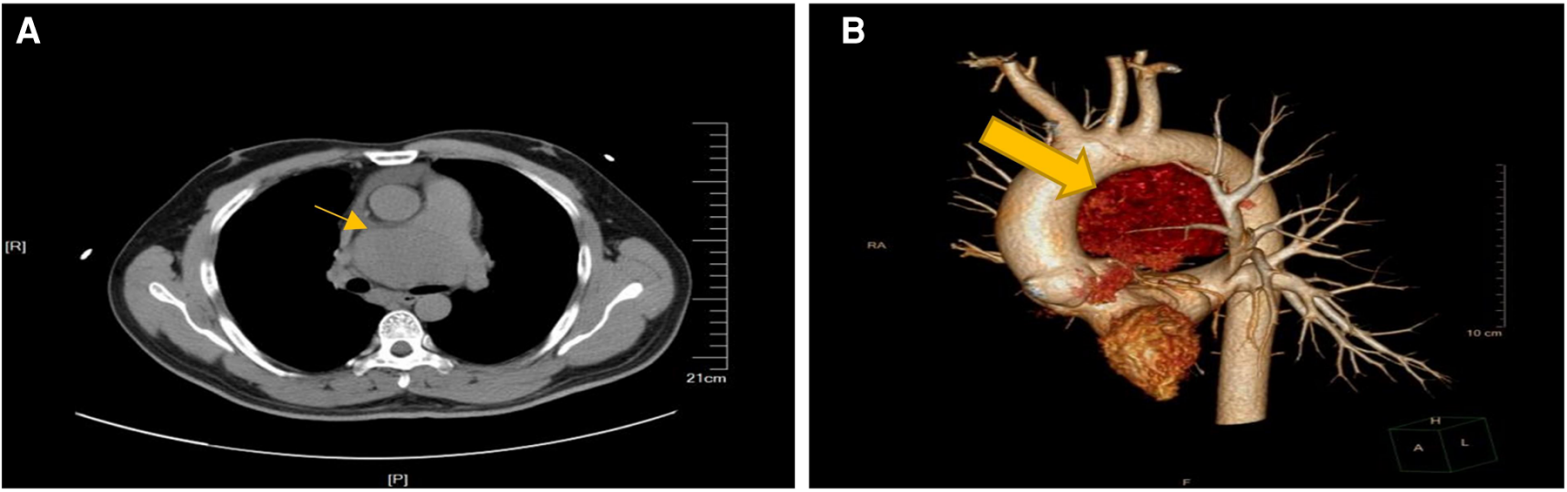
Preparative computed tomography scan of the chest showing in the anterior mediastinum. (A) The mass close to the main pulmonary artery bifurcation and extended along the right pulmonary artery. (B) Lateral view of 3-D computerized tomography showing the mass between aortic arch and pulmonary artery.
